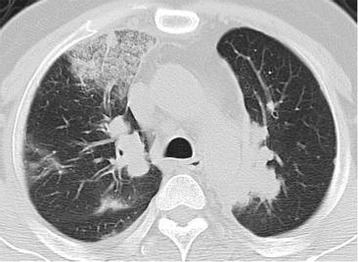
Computed tomography image of nivolumab-induced pneumonitis in Case 2. Multiple consolidations and ground-glass opacities developed at both lungs
